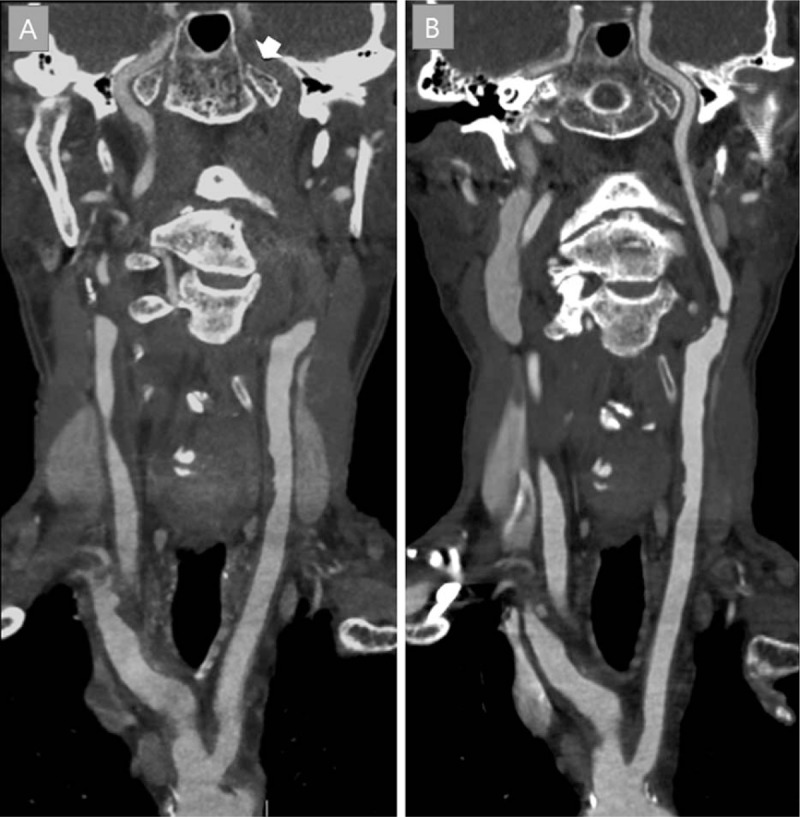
Computed tomography angiography before (A) and after (B) intravenous tissue plasminogen activator. Long segmental occlusion of the internal carotid artery (arrow) is recanalized after intravenous thrombolysis.
